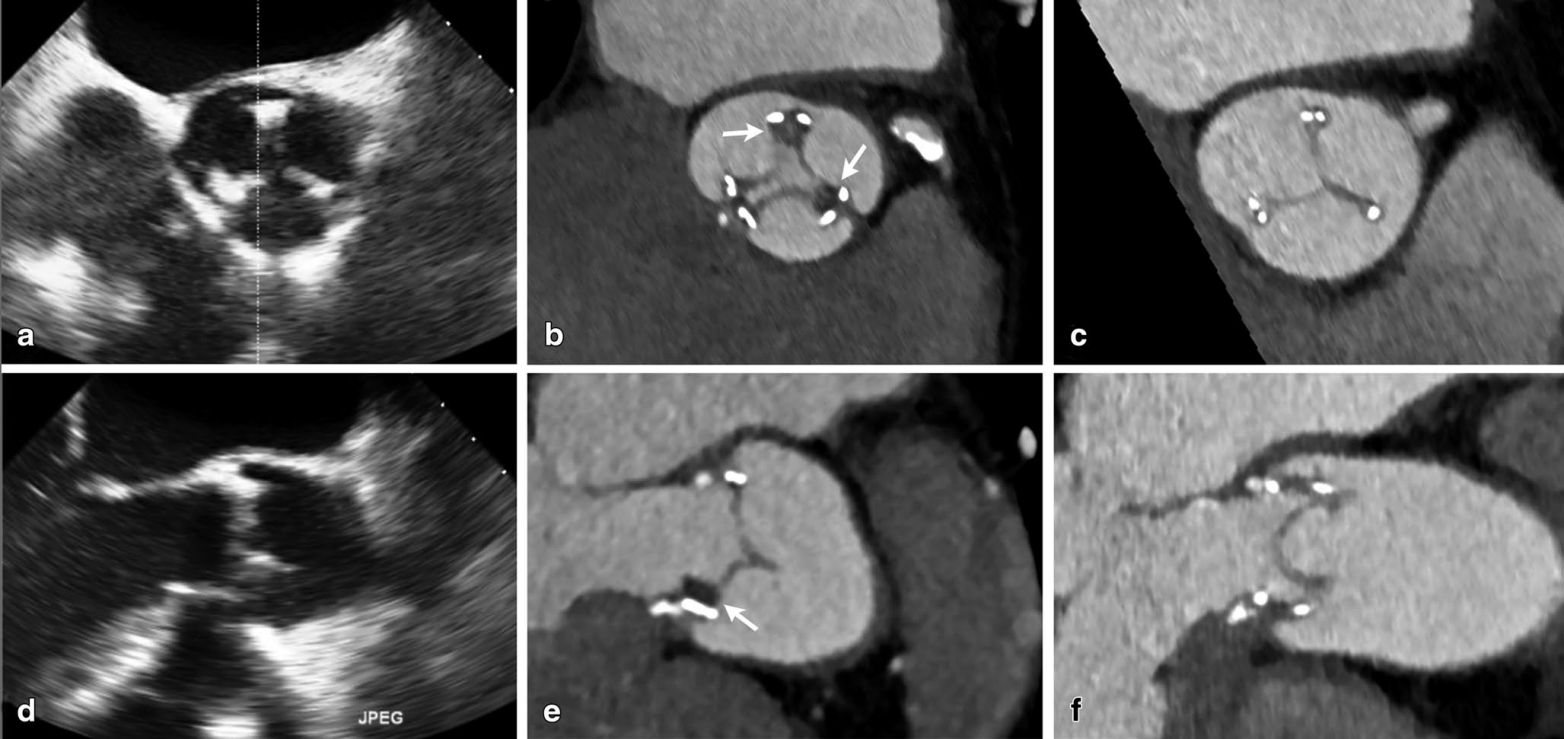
Vegetations missed by TEE. Patient with an aortic bioprosthesis for 3 years, presented with an infection of a knee replacement prosthesis (streptococcus). Blood cultures remained negative and TEE revealed no abnormalities (Panel a and d). CTA however detected hypodense masses (panel b and e, *arrows*) fitting with vegetations, which were successfully treated with 6 weeks of intravenous antibiotic treatment (panel c and f)
